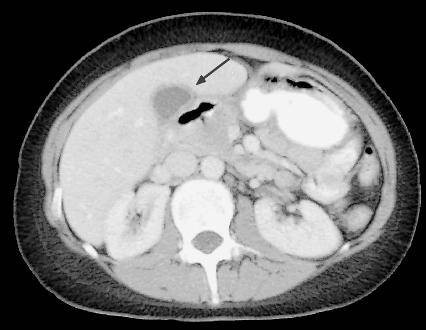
Select axial image of abdominal computed tomography of a 23-year-old white female with a three week history of right upper quadrant pain showing a thickened gallbladder wall (arrow) with surrounding fluid.
